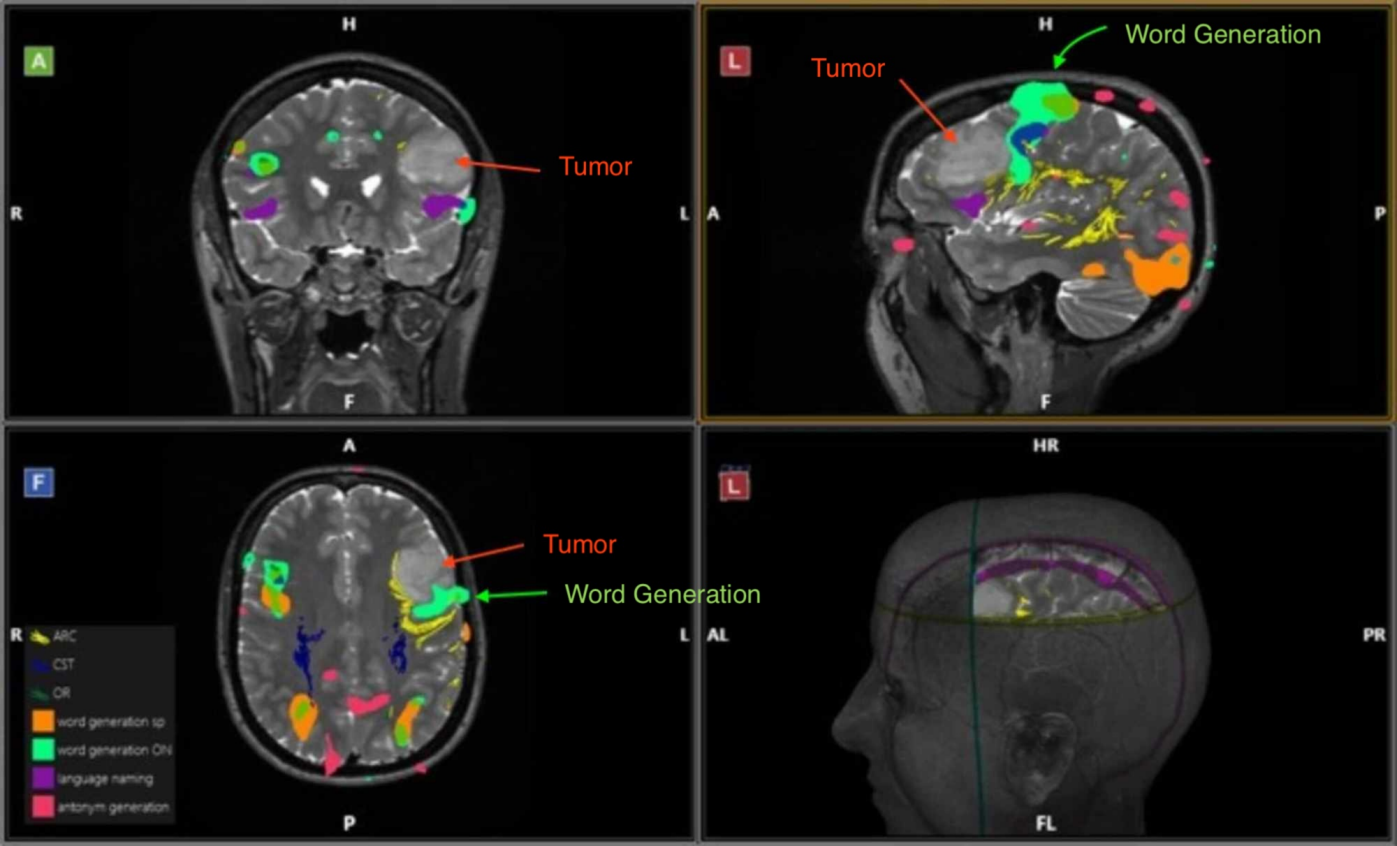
Functional MRI central view. Anterior, lateral, frontal, and lateral perspectives, respectively, show the tumor in the posterior inferior portion of the left frontal lobe, as well as activation for speech (word generation), highlighted in light green, near the inferior portion of the tumor. The tumor tissue is indicated by the red arrow, while the areas of functional activation are indicated by the green arrow.
